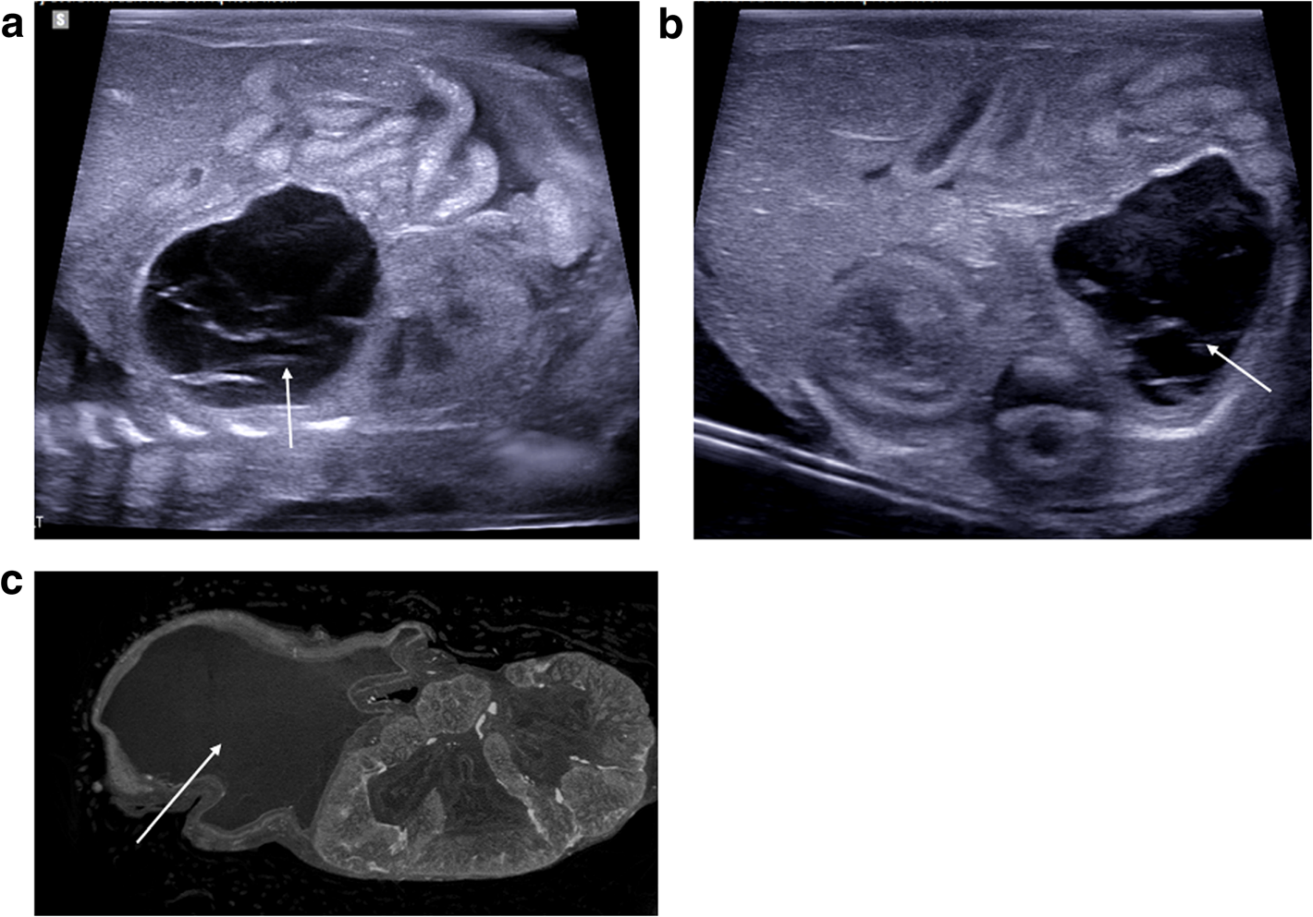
Left adrenal haemorrhage in a foetus at 18 weeks gestation, following termination of pregnancy for megalourethra. The post-mortem ultrasound images of the left kidney in sagittal section (a) and of the adrenal glands in transverse plane (b) were obtained 6 days after delivery. There is a large septated, cystic mass in the left suprarenal space (solid arrows), better depicted on the sagittal contrast enhanced micro-CT imaging (c) of the extracted left kidney. The findings were in keeping with a small haematoma from in utero adrenal haemorrhage
